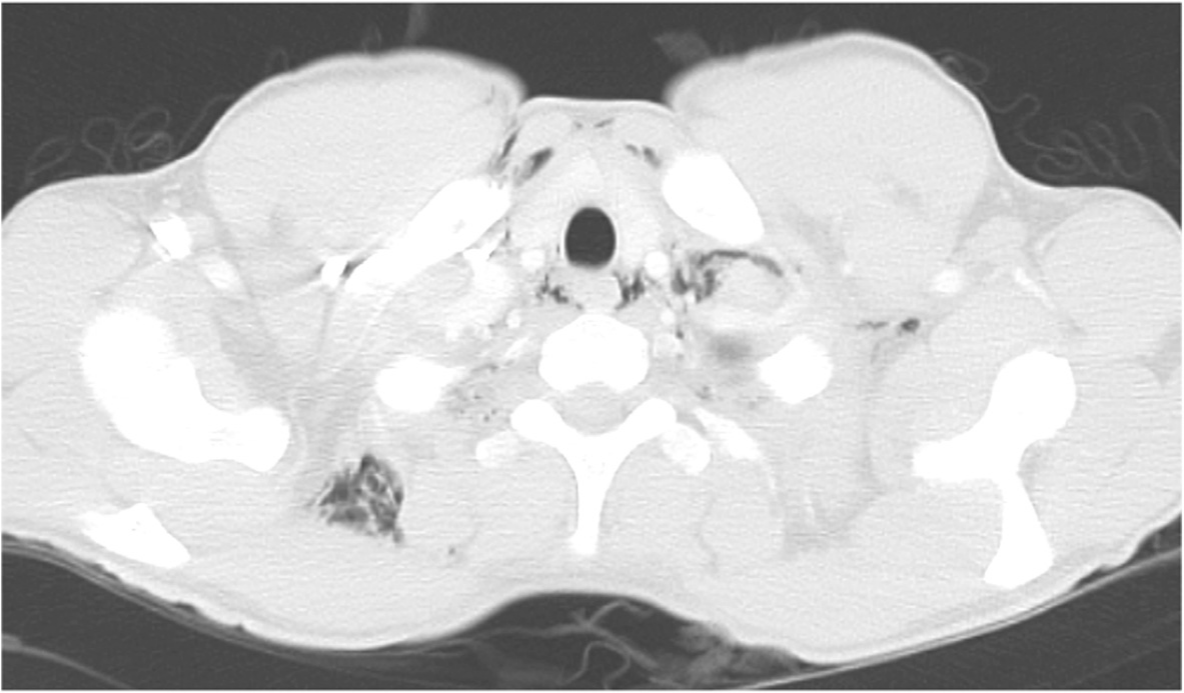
Axial computed tomography showing subcutaneous emphysema in the neck
